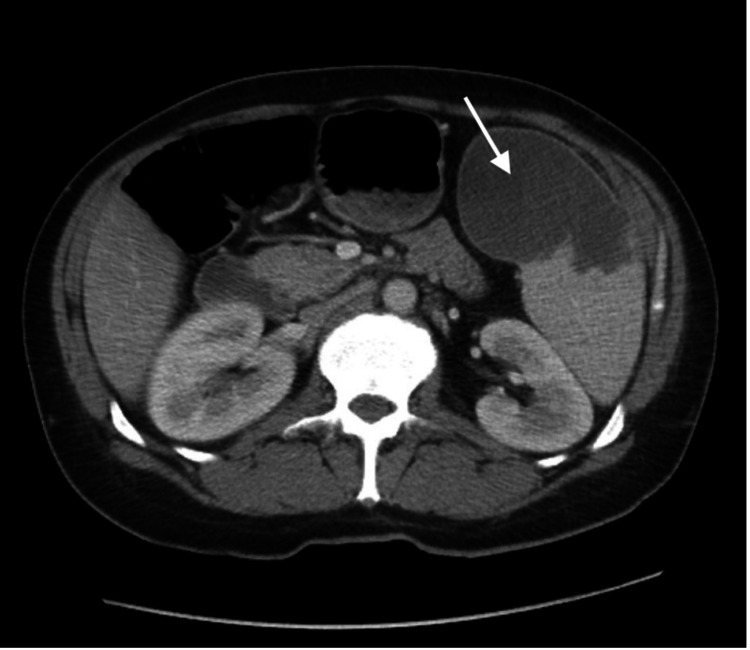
CT of the abdomen and pelvis demonstrating an enlarged spleen with an infarct in the inferior portion of the spleen (arrow)
